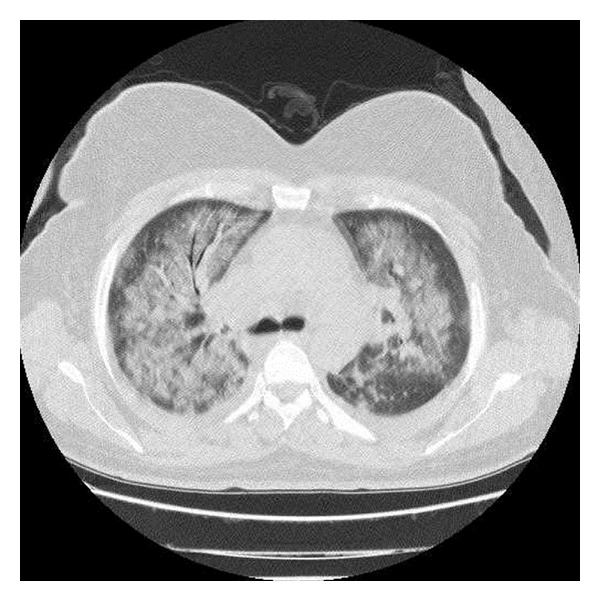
High resolution computed tomography revealed extensive ground-glass infiltrates in both lungs.
